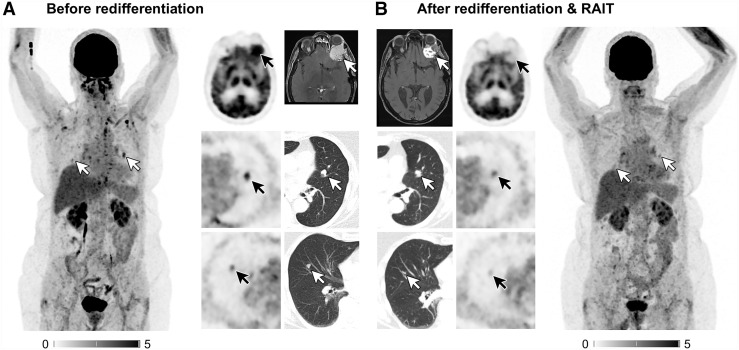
Comparison of glycolytic activity and MRI or CT morphologic course of osseous and pulmonary lesions of patient 7 before (A) and after (B) redifferentiation with consecutive RAIT with significant decrease of [^18^F]FDG uptake and reduction of lesion volumes (only pulmonary lesions and orbita lesion) with assessment of partial response 4 mo after RAIT. hTg level decreased from 590 ng/mL to 53 ng/mL during same time frame in patient 7. Intensity scales are SUV.
